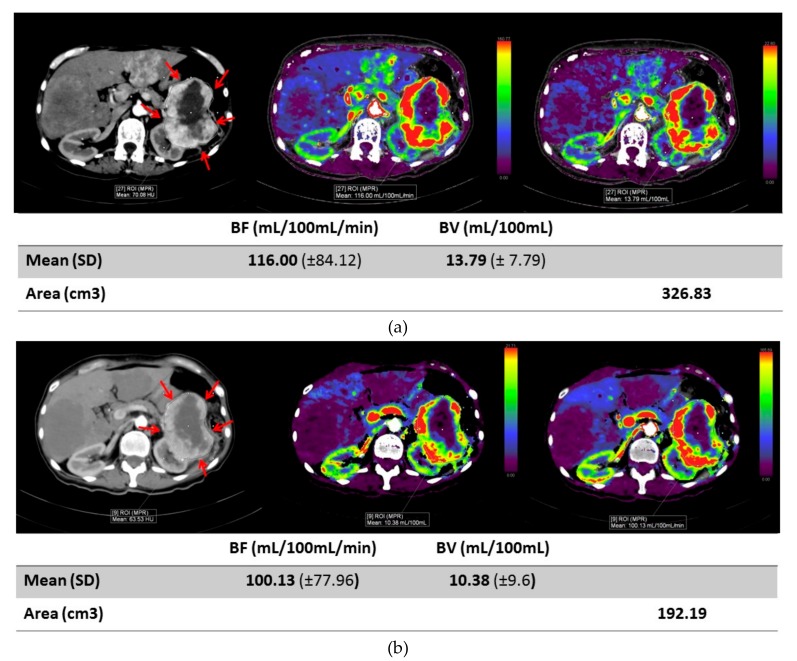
Images of a primary renal lesion. (a) Axial contrast enhanced image and CT perfusion blood flow image before therapy of left renal mass (red arrows). Blood flow was measured to be 116 mL/100 mL/min and blood volume was 13.79 mL/100 mL. (b) An axial contrast enhanced image and CT perfusion image of same renal mass eight days after therapy shows a mild decrease in blood flow to 100.13 mL/100 mL/min and blood volume to 10.38 mL/100 mL, but with stable size of mass. Standard deviation (SD) for each measurement is shown on the table below the figure.
